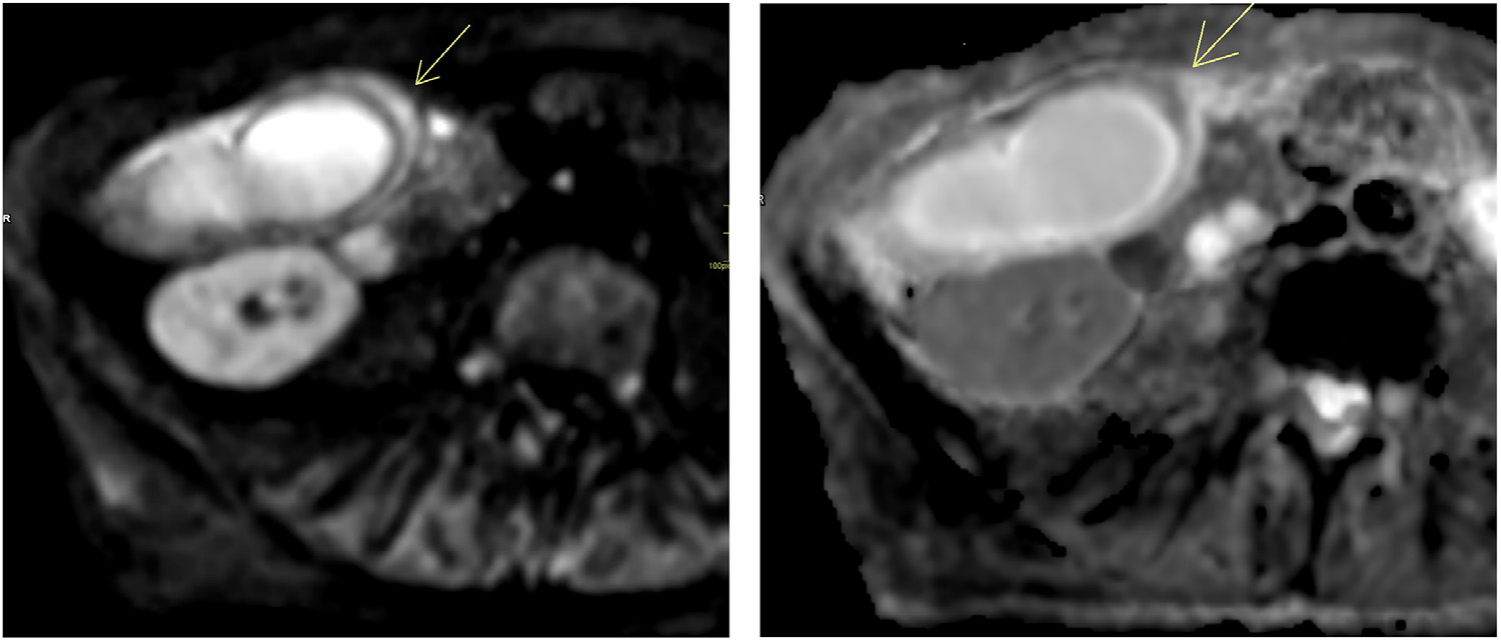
(A) Diffusion-weighted imaging (DWI) sequence (b800) demonstrating pronounced hyperintensity in the gallbladder wall (arrow). (B) Corresponding apparent diffusion coefficient (ADC) map showing diffuse hypointensity, consistent with true diffusion restriction and acute ischemia.
